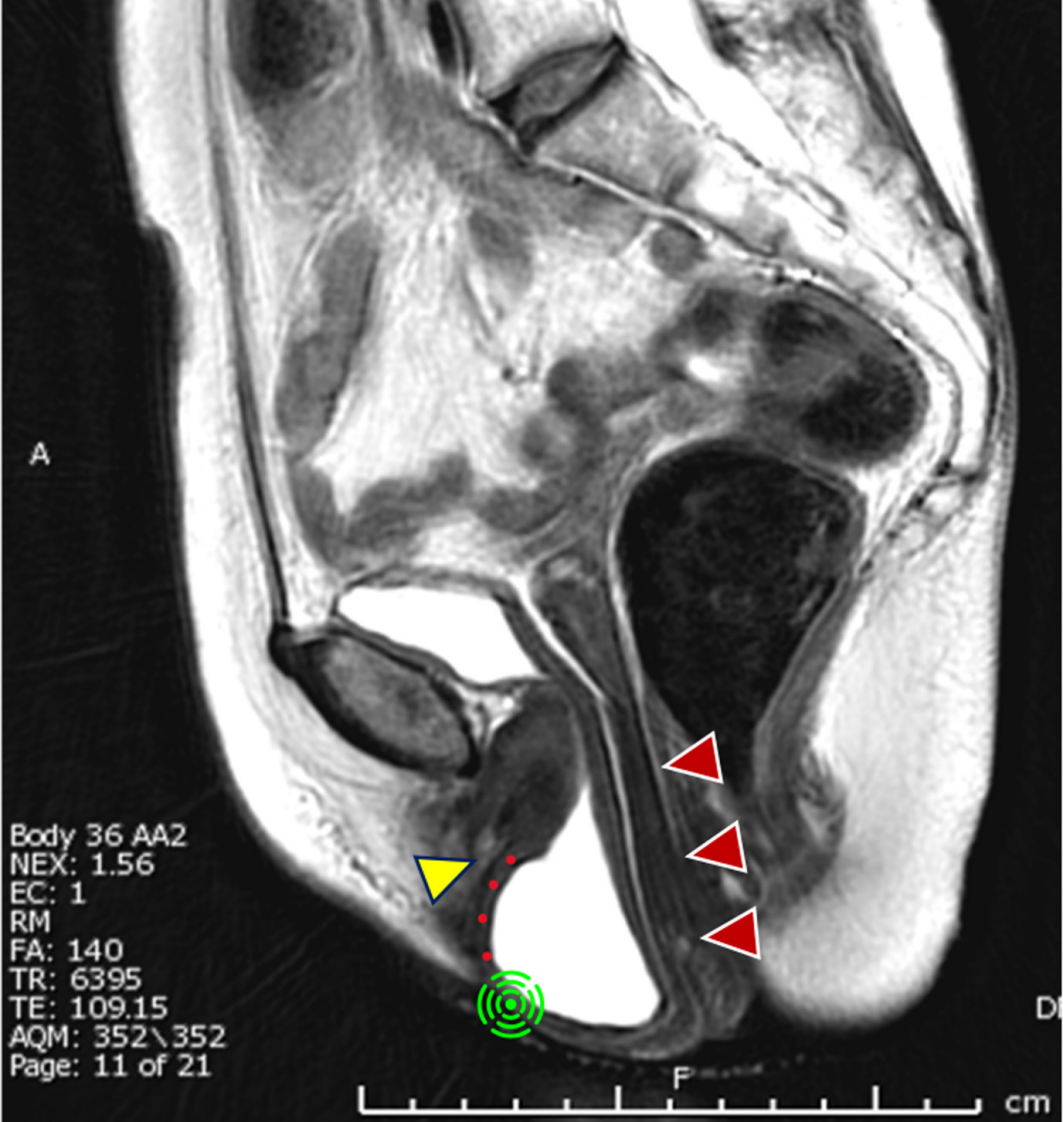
MRI sagittal view. The red arrows indicate uterine cervix and cervical elongation. The anterior vaginal wall, in conjunction with the bladder filled with urine, had undergone a severe prolapse, resulting in a Stage 3 cystocele. The yellow arrow indicates the external urethral meatus. The vaginal point Aa, indicated by a green symbol, was situated 3 cm proximal to the external urethral meatus.
